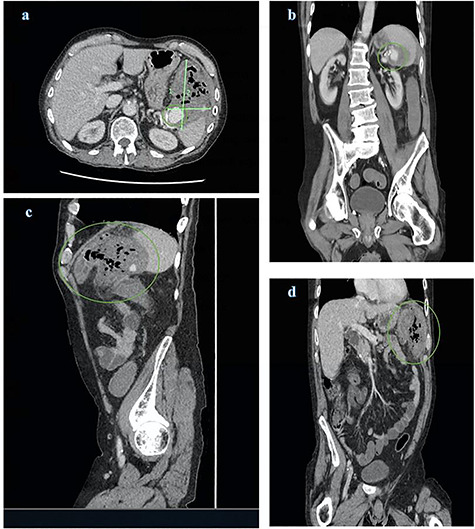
CT abdomen and pelvis views of the known splenic artery pseudoaneurysm with intravenous contrast (a) and (b) surrounded by a large inflammatory mass (a), (c) and (d); the mass demonstrates multiple foci of air and is intimately related to the splenic flexure
